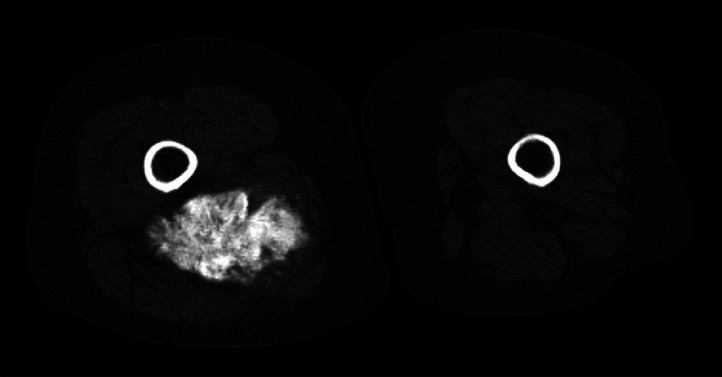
Axial unenhanced CT showing central calcification with ill-defined central mineralization and margins. No contiguity with the underlying femur. No periosteal reaction or aggressive features in the right femur.
